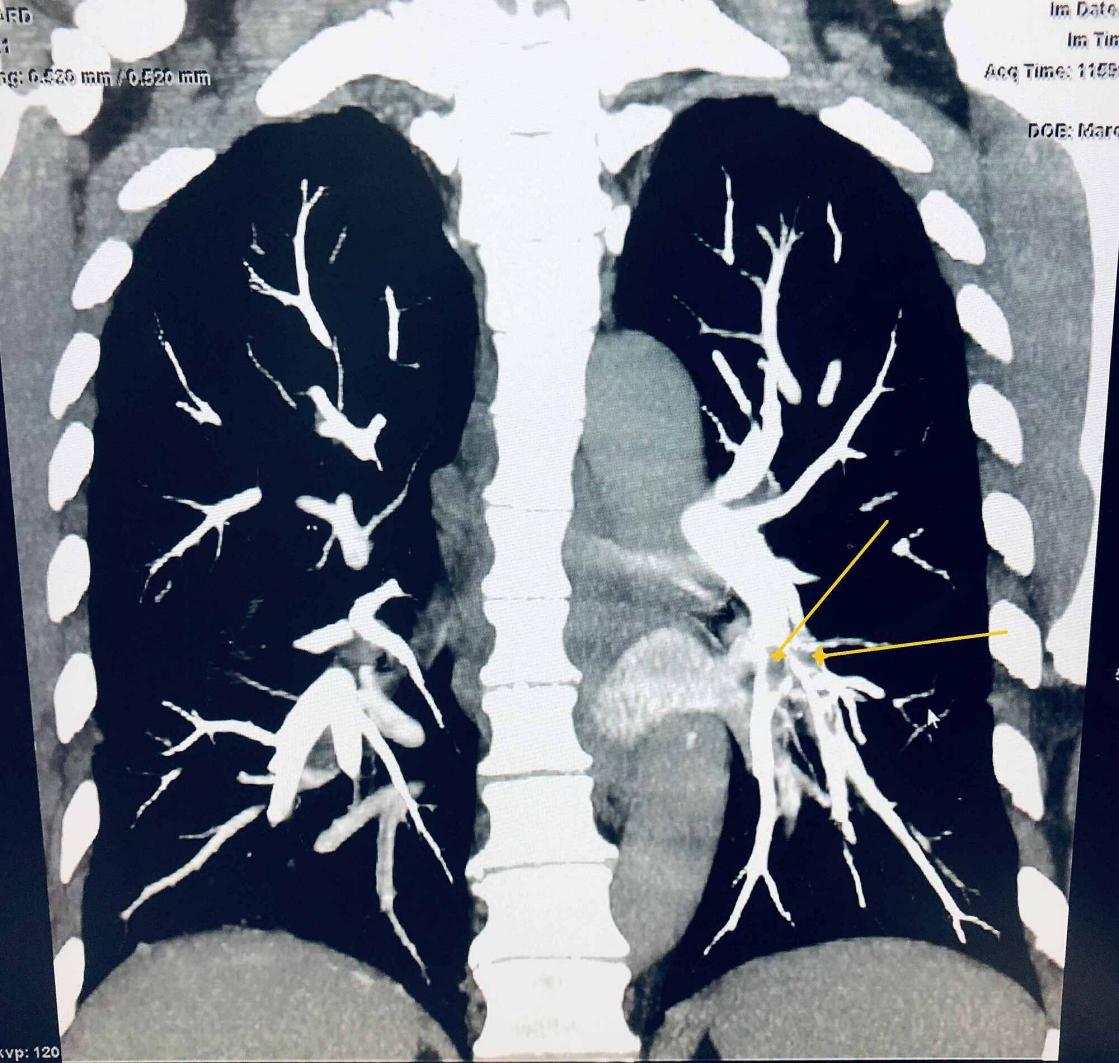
CTA chest coronal view revealing acute appearing pulmonary emboli (pointed by yellow arrows) identified throughout multiple segmental branches of the left lower lobe pulmonary artery. CTA: computed tomography angiography
